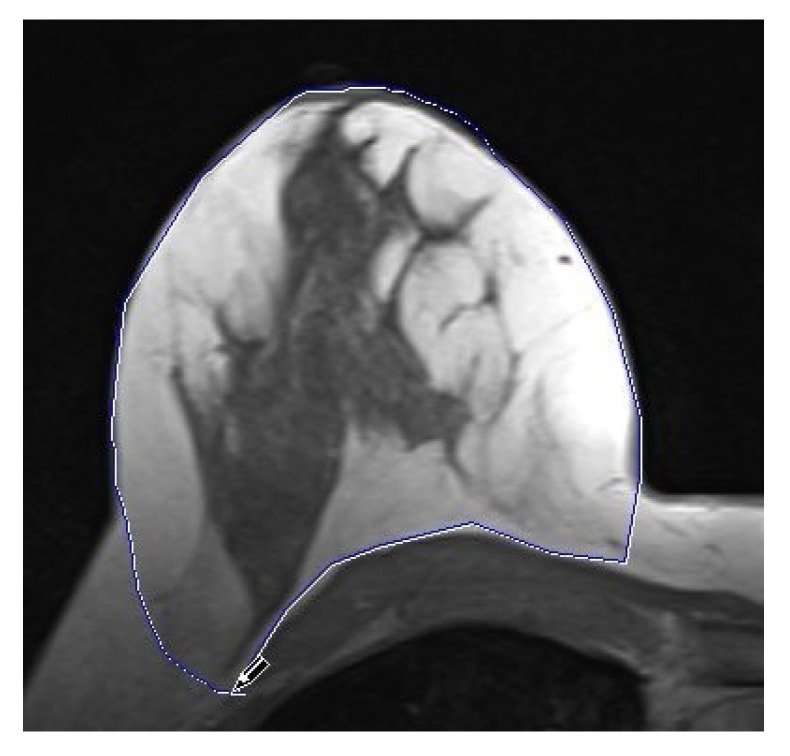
Preoperative volumetry of the whole breast T2-weighted image. The measurements were made using the image processing software Syngo.via (Siemens Healthcare GmbH, Erlangen, Germany).
